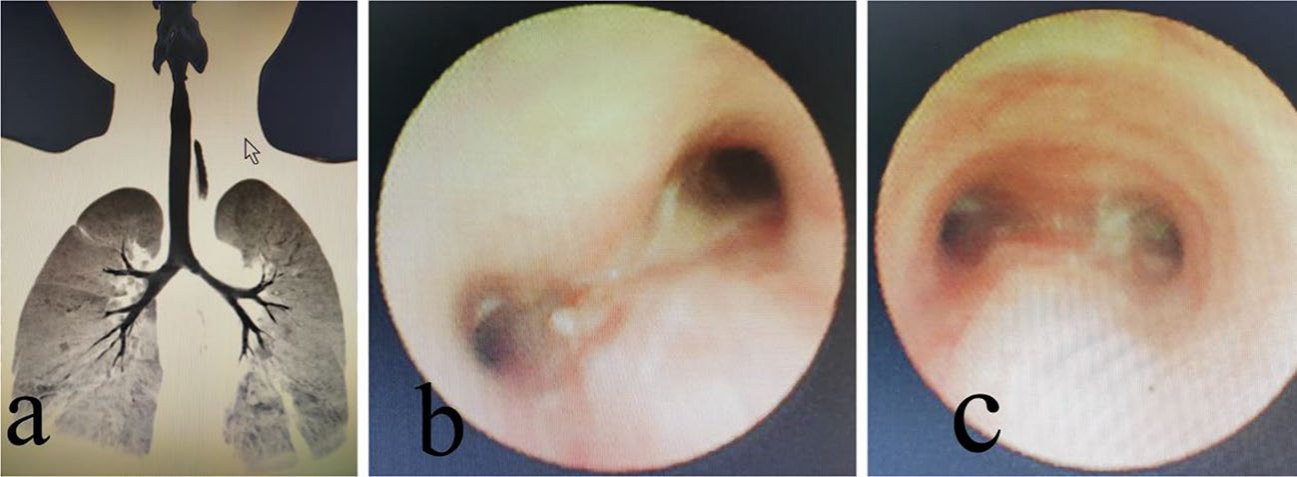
(a) A postoperative coronal CT scan (b) A bronchoscopy image 2 months after surgery (c) A bronchoscopy image 3 months after surgery
